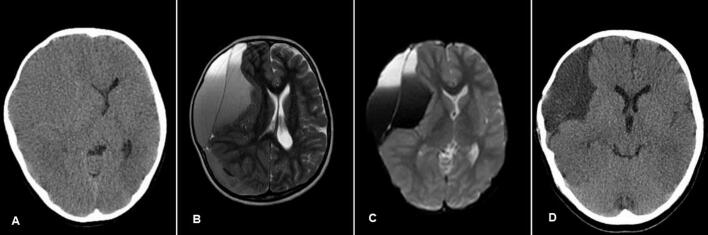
A) Preoperative non-contrast CT scan showing a large isodense lesion in the right frontotemporoparietal region, suggestive of an arachnoid cyst with possible hemorrhagic content. B) Preoperative MRI (T2-weighted) demonstrating a double-layered Sylvian arachnoid cyst with significant mass effect and compression of adjacent brain structures. The presence of fluid-fluid levels is indicative of subacute hemorrhage within the cyst. C) Another preoperative MRI revealed the tense outer cystic wall and internal hemorrhage with a mild displacement of midline structures. D) Postoperative non-contrast CT scan (6 months after surgery) showing significant reduction in cyst size with resolution of mass effect.
